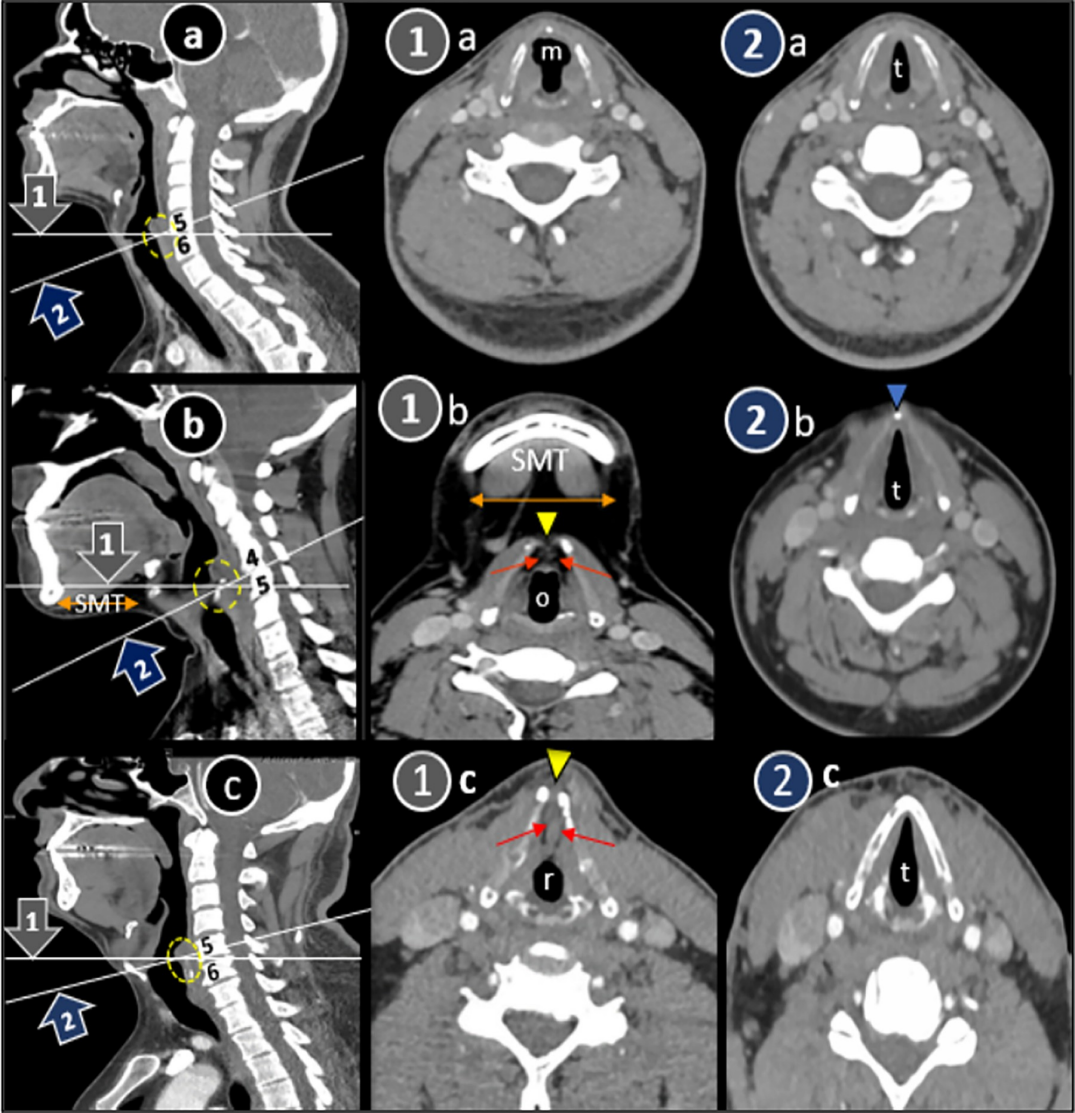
Sagittal post-contrast CT scan neck (a-c) showing two axial CT scan plane lines, non-parallel① and parallel to vocal cord ② intersecting at level of cricoarytenoid joint (dotted circle). Altered glottic parameters in plane① (1a-1c) are shown i.e., change in glottic lumen shape from tear (t) to mushroom (m), oval (o) and round (r), open and close thyroid laminae (yellow and blue arrowheads), inclusion of submental triangle (SMT, horizontal double head arrows) and adipose tissue (red arrows). Crossing of two axial planes (①,②) through cervical vertebrae (4,5,6) are also shown. Parallel plane② CT scans are also displayed for comparison (2a-2c).
